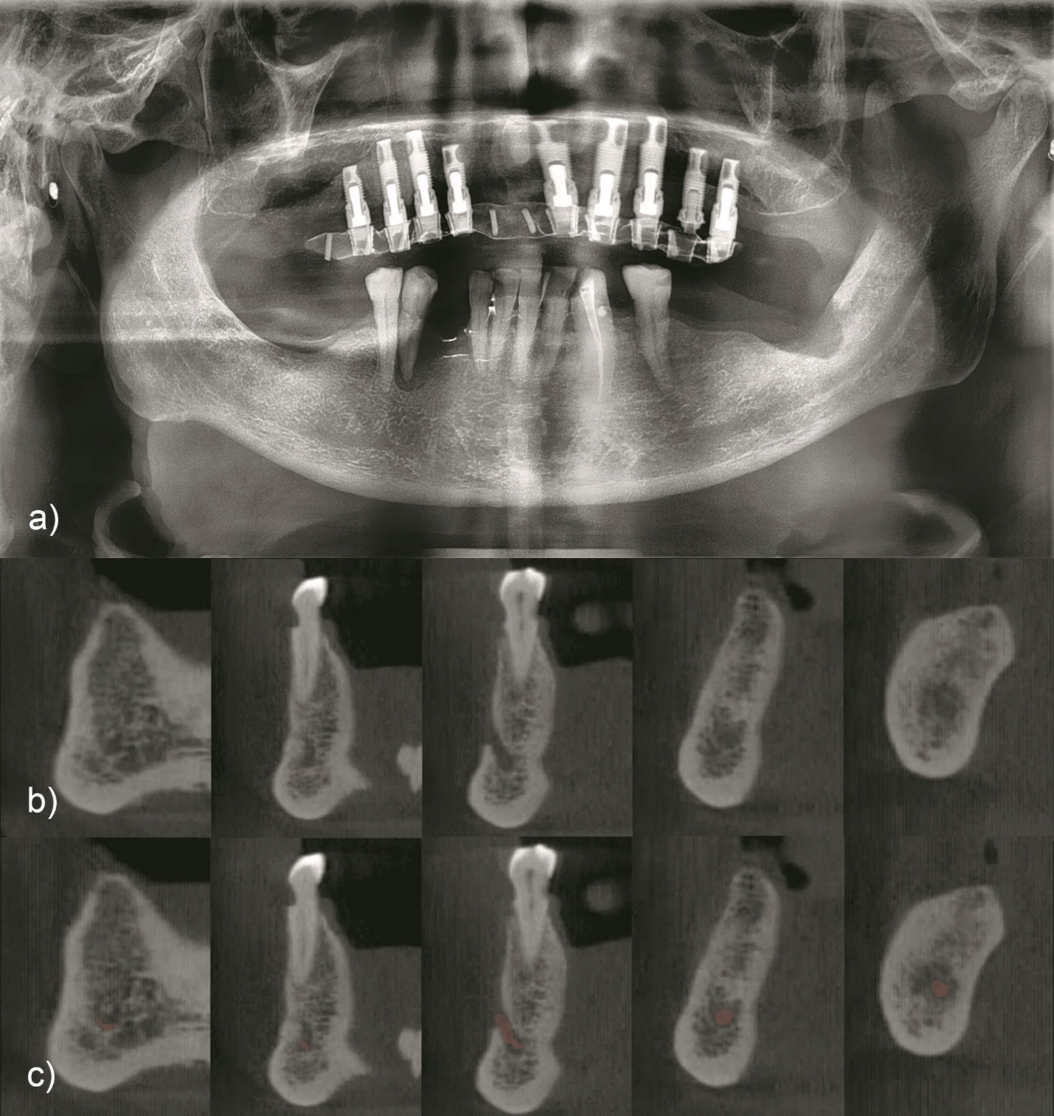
Case of 81-year-old male. (a) Panoramic radiograph. (b) Cross-sectional
